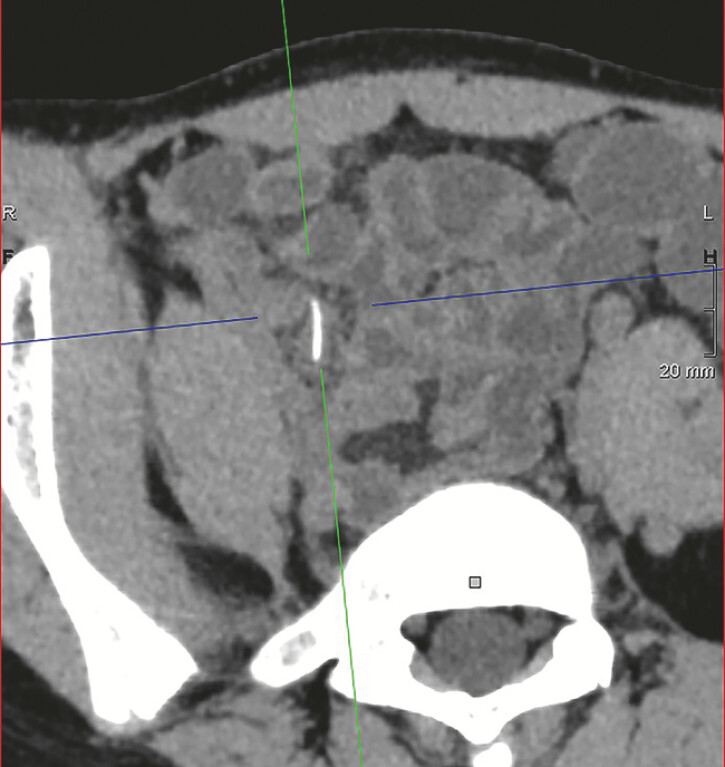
A computed tomographic image showing a high-density shadow in the right lower quadrant of abdomen, indicating an intestinal foreign body.
